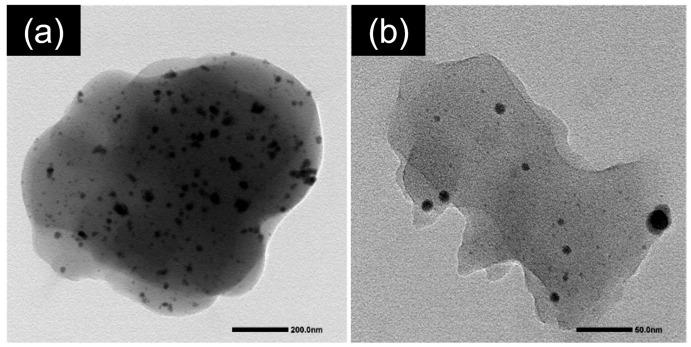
TEM images of particles extracted by ultrasonication from cotton fabrics surface-modified with (a) Cot-αCS-L-Ag and (b) Cot-βCS-L-Ag.
